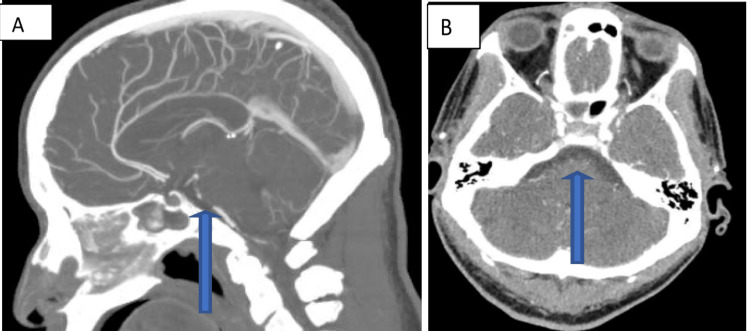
(A and B) Sagittal and axial views of CT angiography show short-segment complete occlusion of the proximal basilar artery with distal reconstitution (blue arrows).
